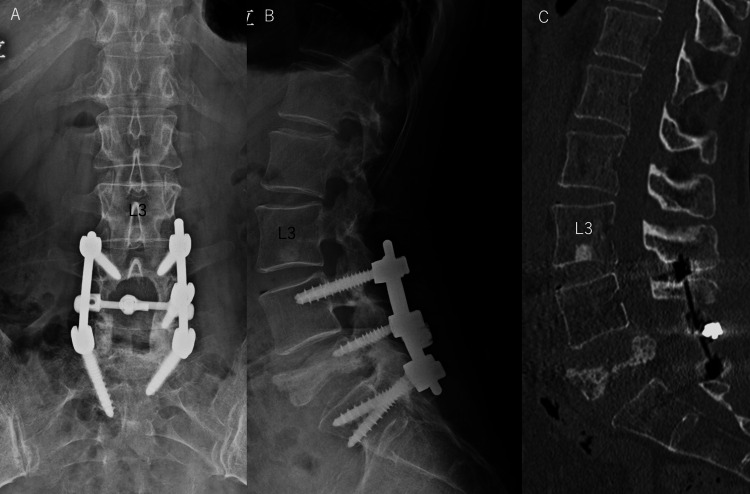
(A) A plain lumbar spine anteroposterior X-ray image at one year postoperatively. (B) A lateral plain lumbar spine X-ray image at one year postoperatively. (C) Sagittal CT image at 11 months postoperatively. A. There was no implant loosening, and good alignment was well maintained. B. Sclerotic change of L5 vertebral body was revealed, and good alignment was well maintained. C. Sclerotic change of L5 vertebral body was confirmed, suggesting well tumor control, and the spinal canal was enlarged.
